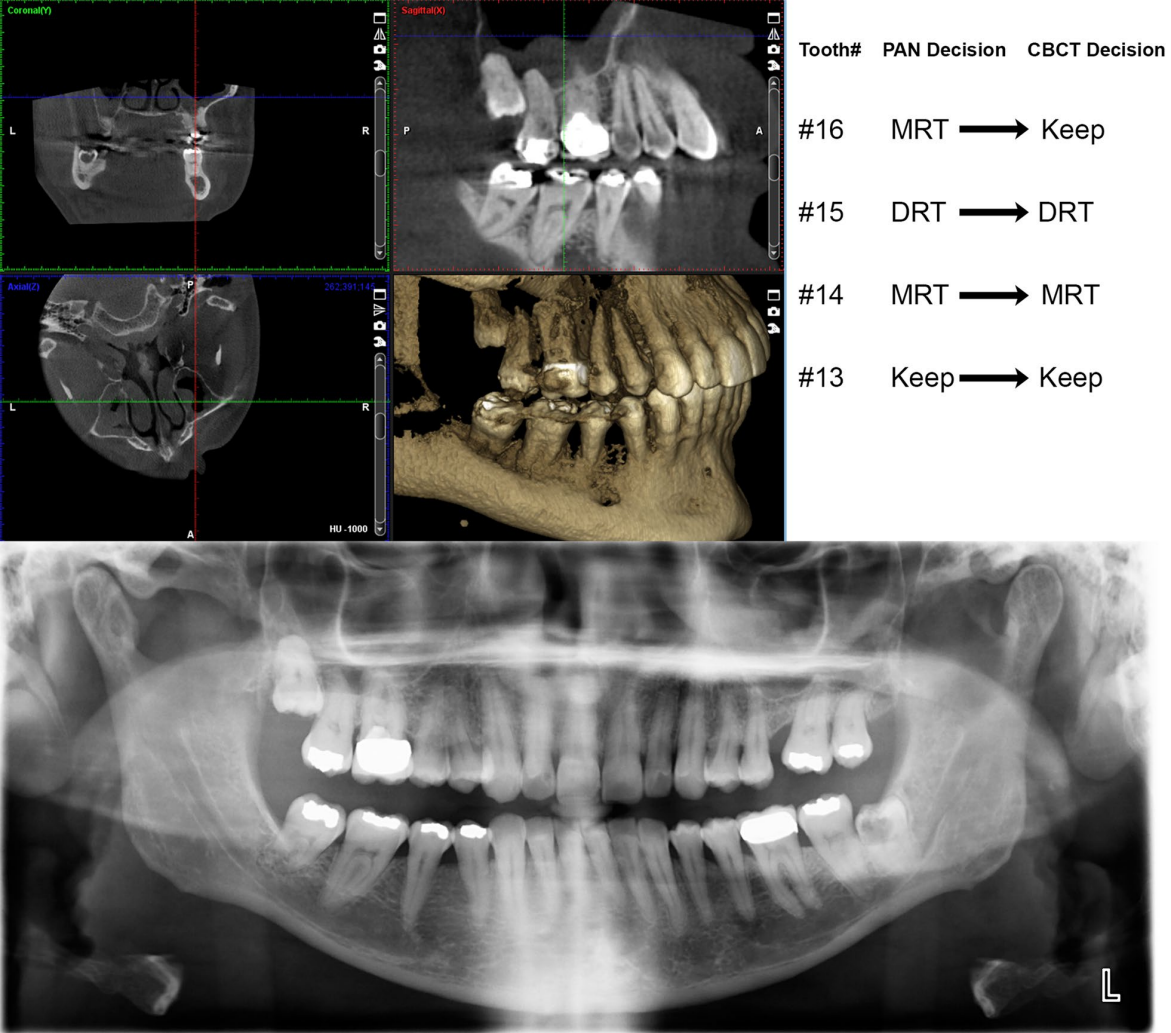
An example of repositioning decisions made by examiner 1 for the upper right quadrant of displayed CBCT and PAN images are shown on the upper right portion of the figure. The CBCT view displaying right side teeth with focus on the upper right quadrant is shown on the top left side of the figure. The corresponding PAN image is displayed on the bottom of the figure
